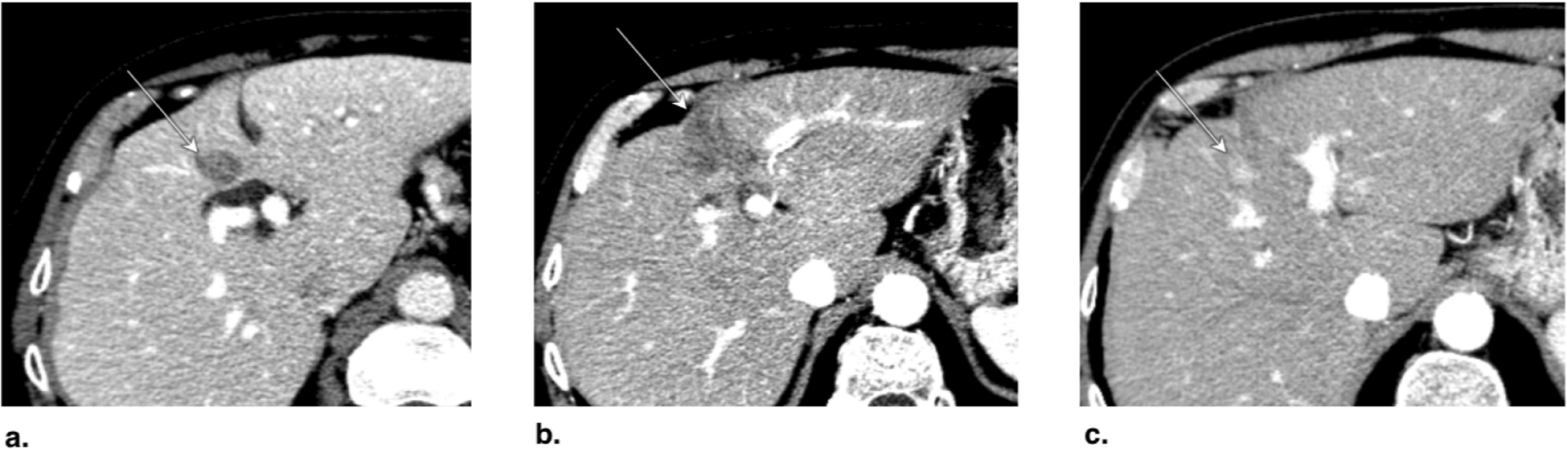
a Axial CT in portal venous phase obtained pretreatment: image shows washout of a 2.5-cm LR-5 observation. Proximity to gallbladder and biliary bifurcation contraindicates radiofrequency ablation. Axial CT in late arterial phase obtained 1 month post percutaneous ethanol ablation: images show (b) hypoenhancement of the treatment area (arrow), with (c) nodular arterial phase hyperenhancement at the upper margin of the treated lesion indicating viable tumor (arrow). The treated observation is categorized LR-TR Viable
